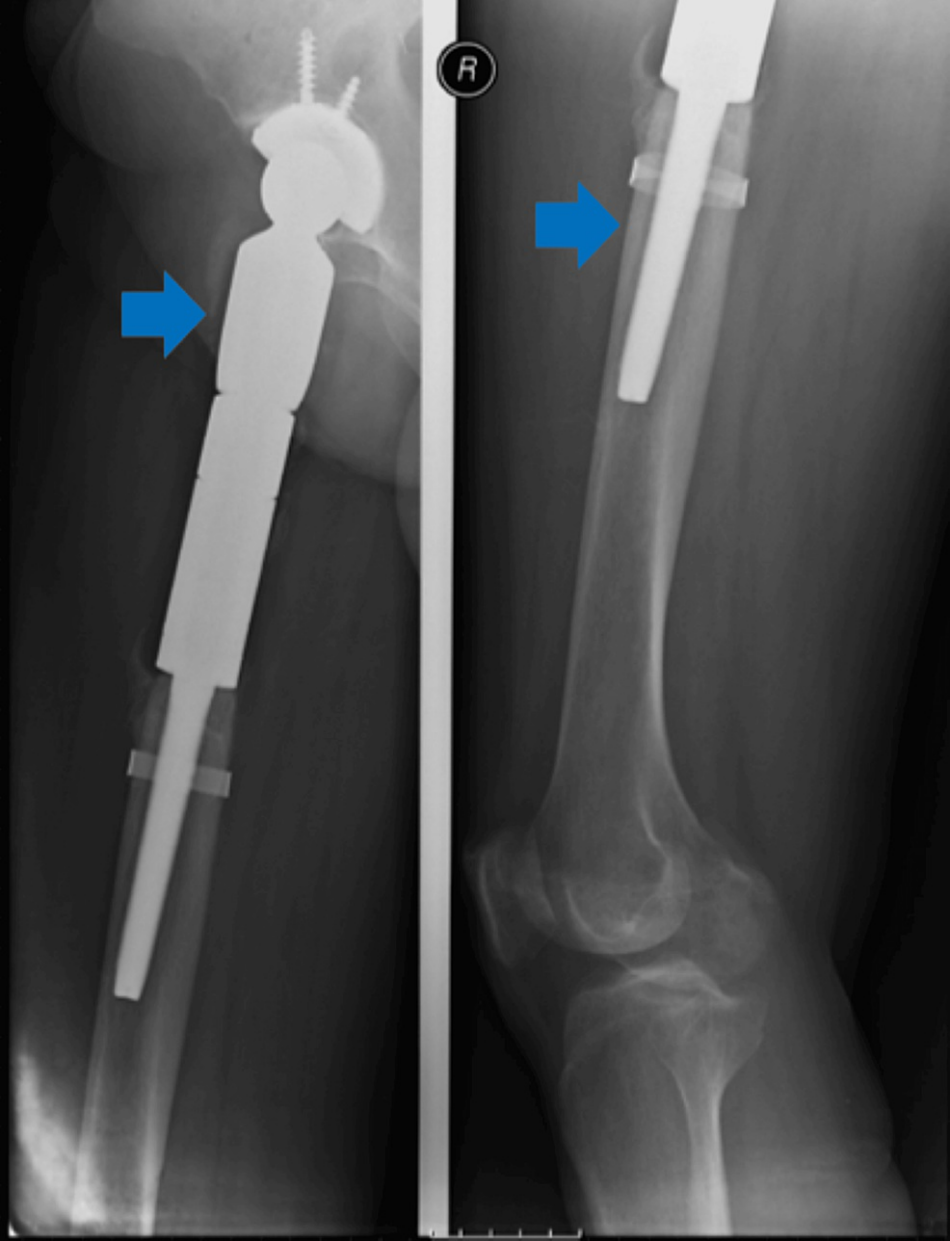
The right hip plain radiograph after orthopedic surgery Blue arrows indicate a modular mega prosthesis in the right hip
